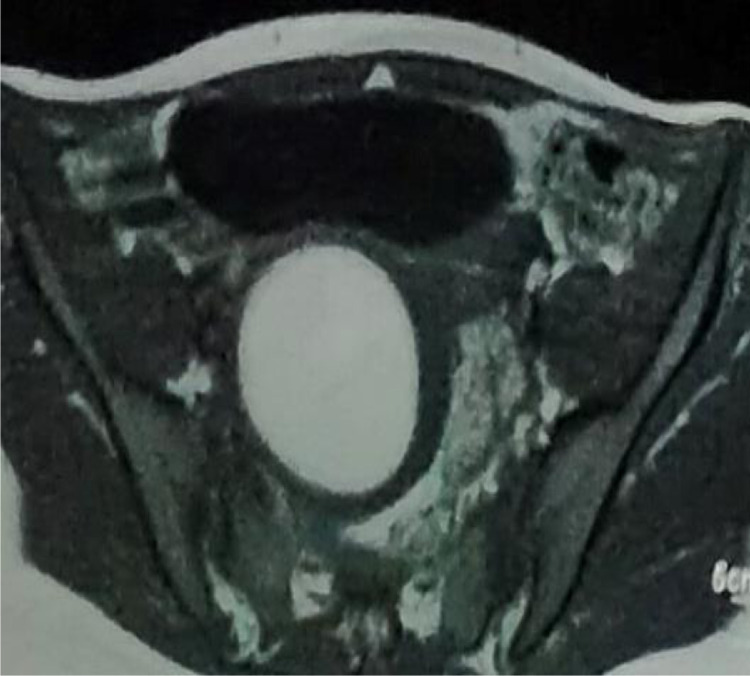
Axial MRI pelvis demonstrates hematocolpos with compressed leftt hemivagina.
